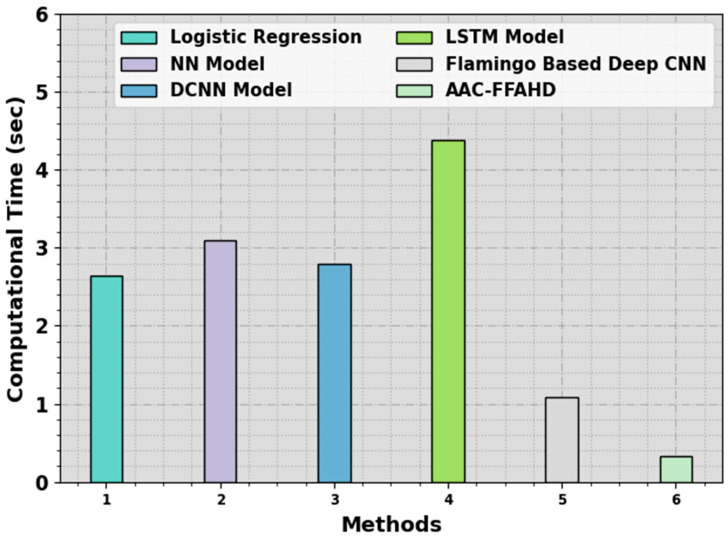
CT outcomes of the AAC-FFAHD system and other methods.
